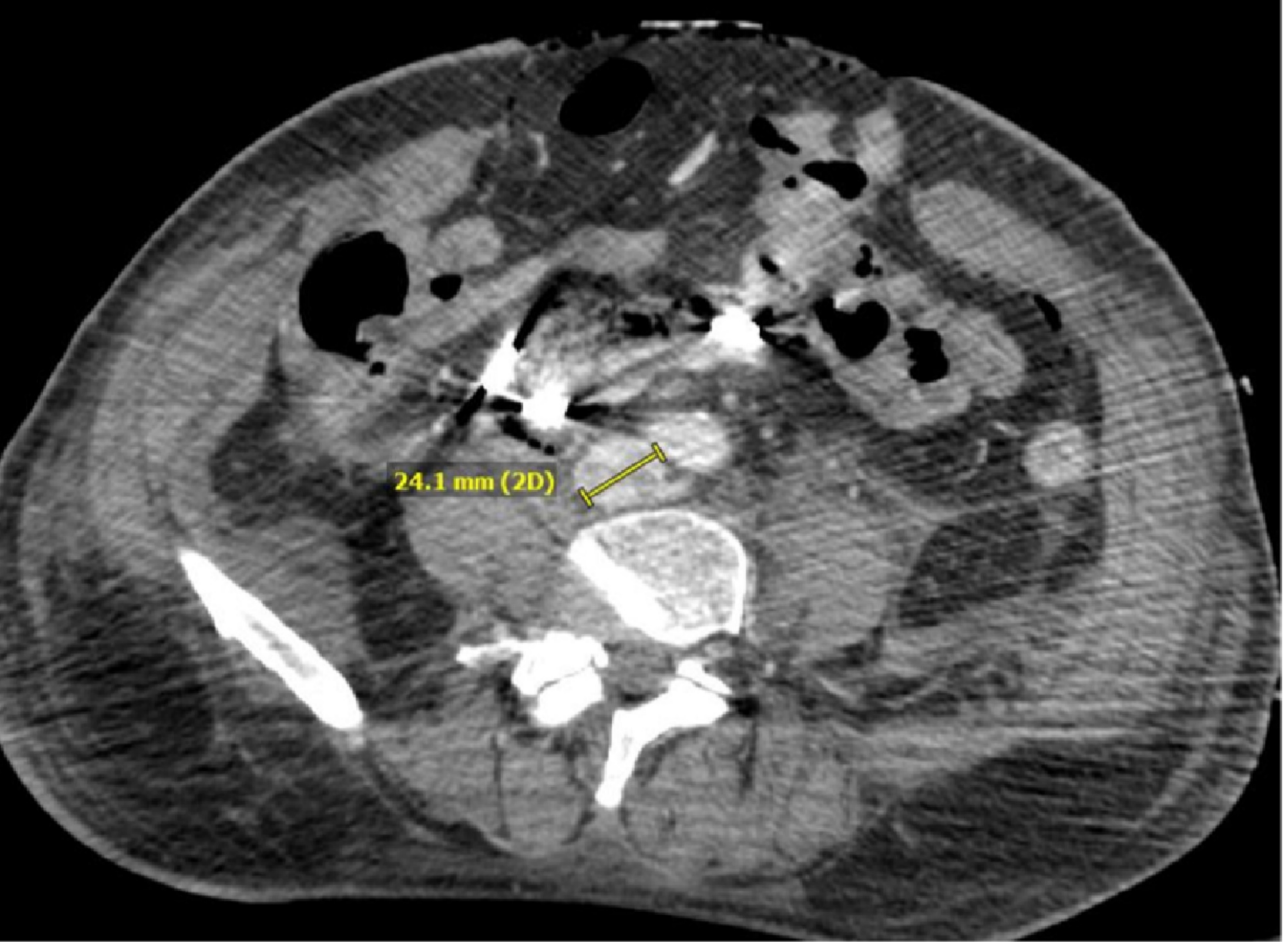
Axial CT of the measured inferior vena cava An axial CT of the patient's measured inferior vena cava is shown, with the diameter being 24.1 mm. This is considered the native vessel diameter and can be used to calculate the side-by-side iliac stent diameters needed for adequate bifurcation repair. CT: computed tomography; IVC: inferior vena cava
